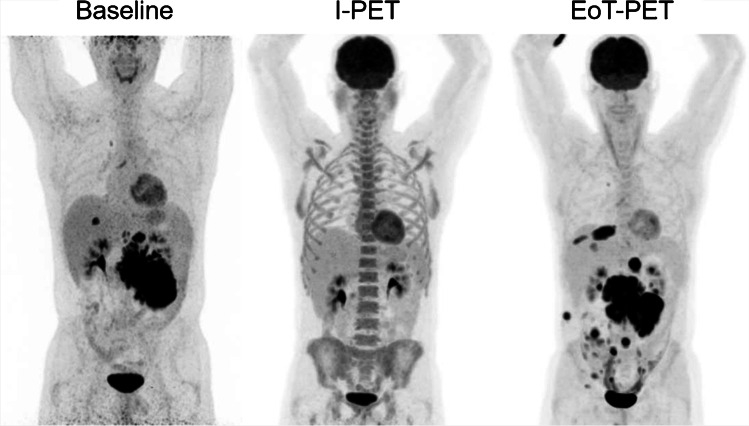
Maximum intensity projections of a *MYC* + DLBCL patient that presented with new PET positive lesions at EoT-PET that were not initially involved. Images are scaled using a SUV0-10 scale
